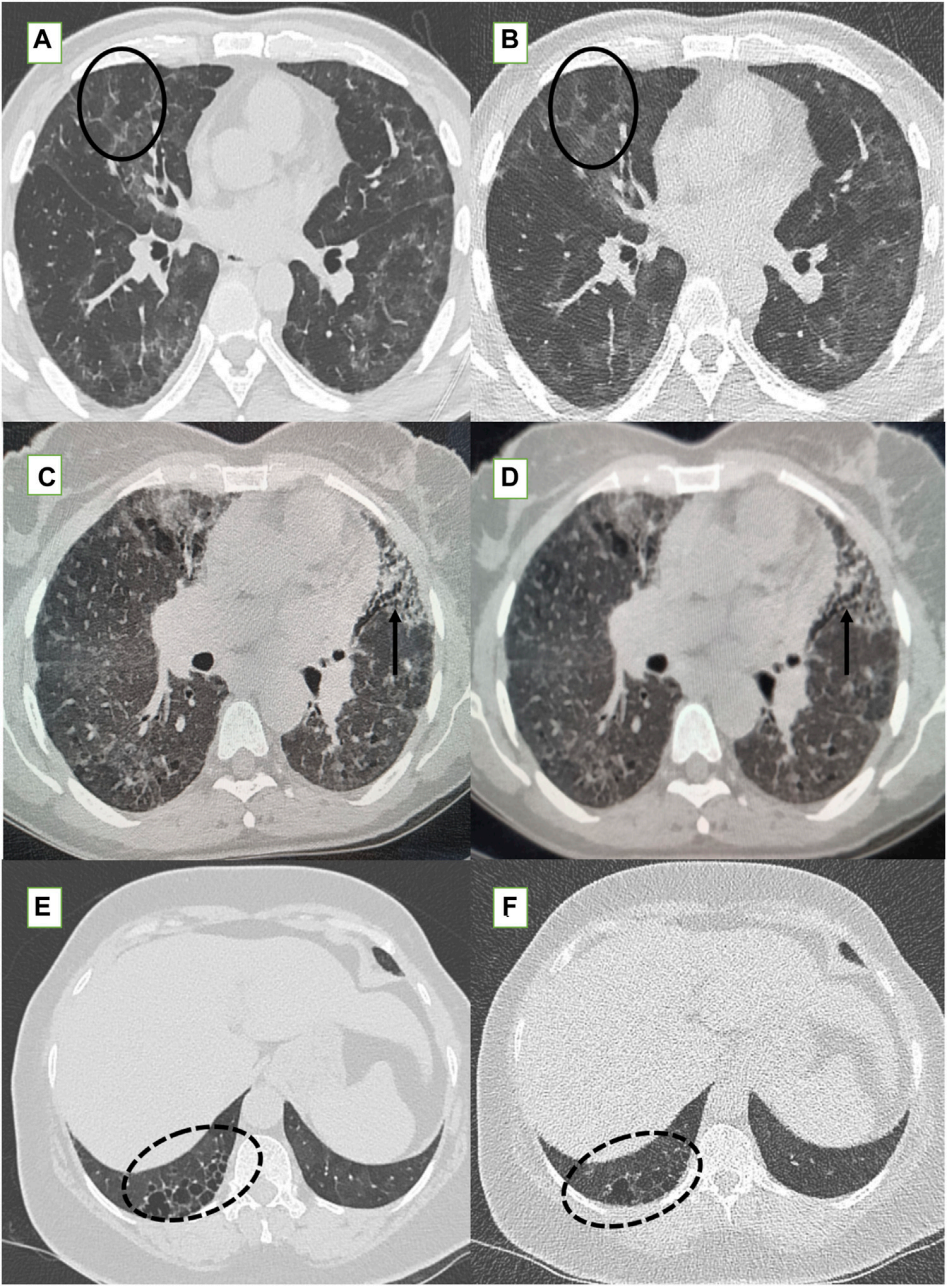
Standard-dose CT (SDCT) chest (left column) and corresponding ultra-low dose CT (ULDCT) chest images (right column) in three different patients: (A,B) 47-years old male long COVID follow up patient presented with fatigue and cough. SDCT chest (A) and corresponding ULDCT chest (B) showing patchy GGOs, septal thickening and mosaic attenuation (black elliptical circle) in both lungs. Effective radiation dose for SDCT and ULDCT was 4.3 mSv and 0.26 mSv respectively. (C,D) Another 56-years old female long COVID follow up patient presented with cough. SDCT chest (C) and corresponding ULDCT chest (D) showing tractional bronchiectasis (small black arrows) along with changes of sub-pleural cysts. Effective radiation dose for SDCT and ULDCT was 4.7mSv and 0.23 mSv respectively. (E,F) A 56-years old female long COVID follow up patient presented with dyspnea and cough. SDCT chest (E) and corresponding ULDCT chest (F) showing honeycombing in RLL (dotted black elliptical circle). Effective radiation dose for SDCT and ULDCT was 3.9 mSv and B-0.22 mSv respectively. CT severity score calculated on both SDCT and ULDCT was similar in all three patients.
